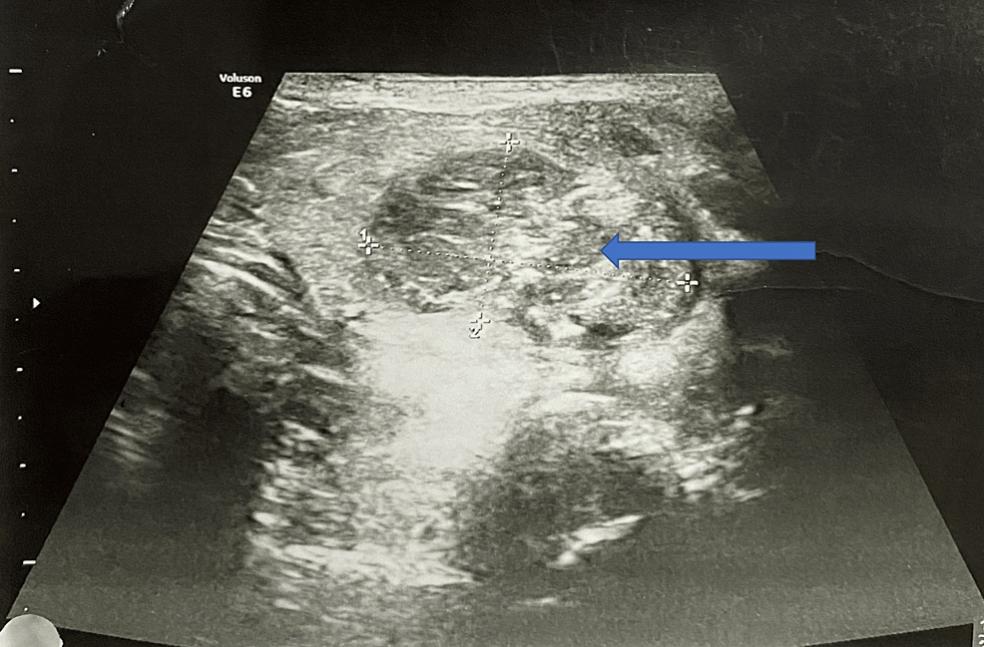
Ultrasonography image of neck/thyroid Ultrasonography of neck/thyroid showing heterogeneous nodule measuring 28 × 18 mm in the left lobe with internal calcification (shown in blue arrow). The left thyroid lobe appears to be heterogeneous with increased vascularity.
